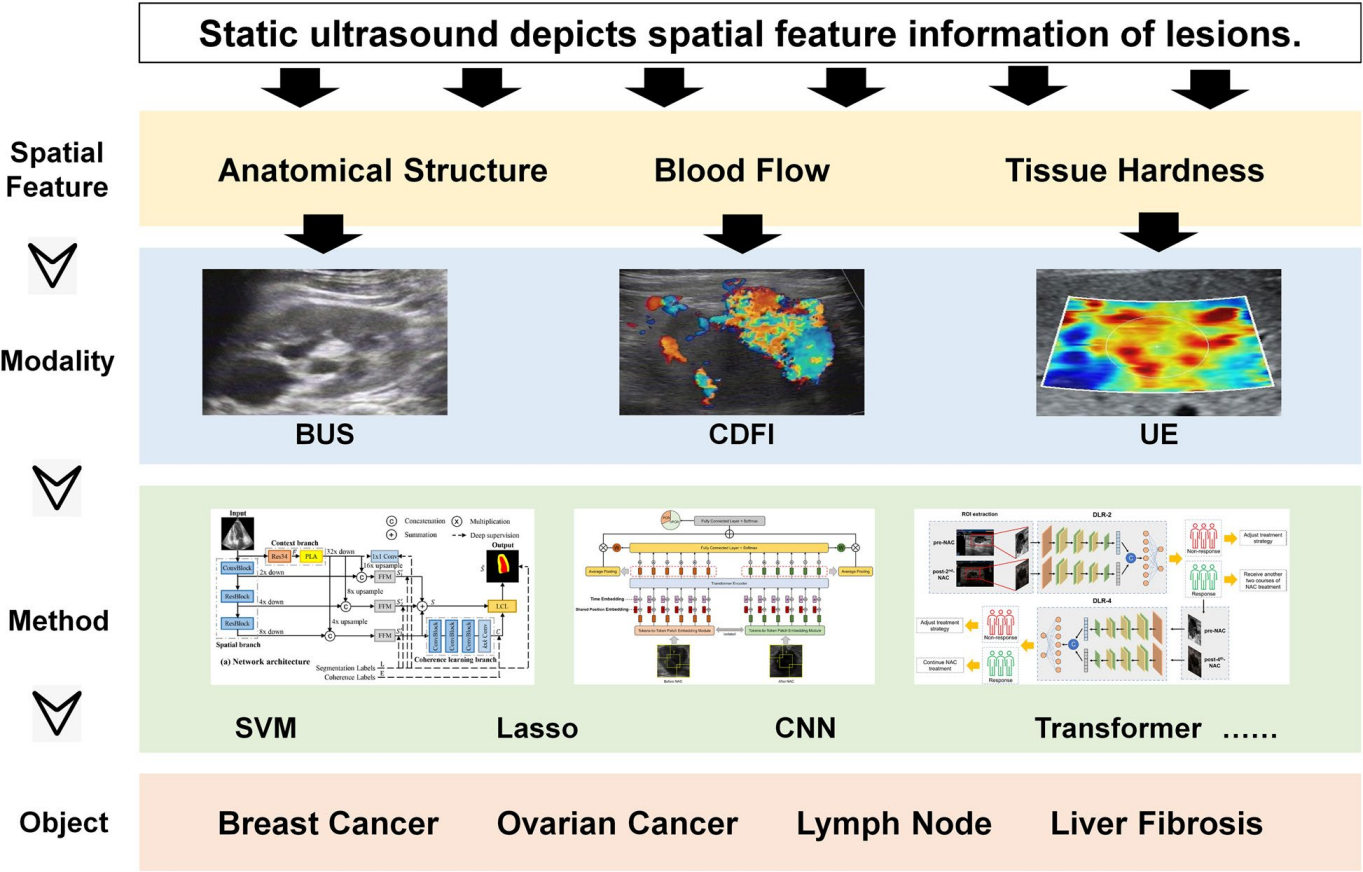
Static ultrasound analysis model for diagnosis and prediction. Static ultrasound reflects the spatial characteristics of lesions. It involves two-dimensional imaging. The BUS images provide information on the anatomical structure, shape, texture, and position of lesions. Moreover, the CDFI images reveal the direction of blood flow in the lesions. Additionally, UE images reflect the tissue hardness of the lesions. The existing intelligent analysis methods for static ultrasound include SVM, lasso regression, CNNs, and transformers. These are commonly used for the diagnosis and prognosis of diseases such as breast cancer, ovarian cancer, lymph nodes, and liver fibrosis
